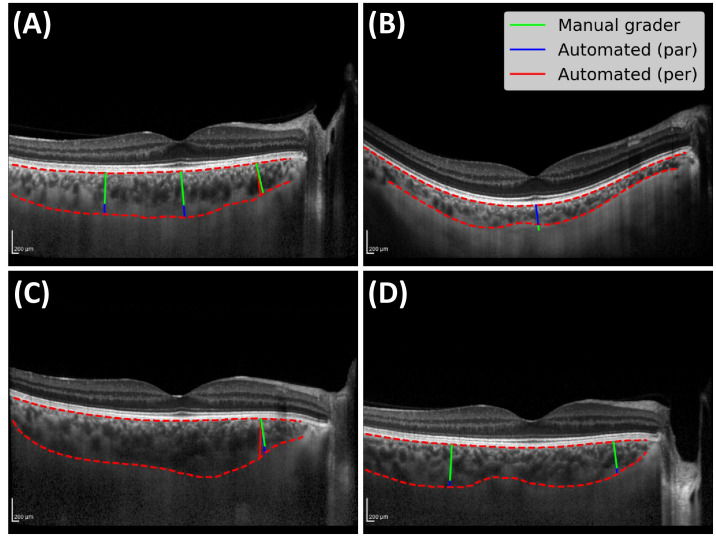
(A) A selection of major discrepancies between automated and manual CT measurements. *Red lines* represent perpendicular automated CT, *blue* represent parallel automated CT, and *green* represent manual CT. The lines in *red* are shown in (A, C) to demonstrate the observed angular deviation between perpendicular automated CT and manual CT. (A) Temporal and subfoveal major discrepancies from a donor, residuals 97 µm and 114 µm, respectively. Nasal angular deviation (5.4°) resulted in perpendicular and parallel residuals as 22 µm and 2 µm, respectively. (B) Subfoveal major discrepancy from a recipient, residual –47 µm. (C) Nasal angular deviation (8.1°) shows perpendicular and parallel residuals as 106 µm and 79 µm, respectively. (D) Temporal and nasal major discrepancy from a donor, residuals 66 µm and 65 µm, respectively.
